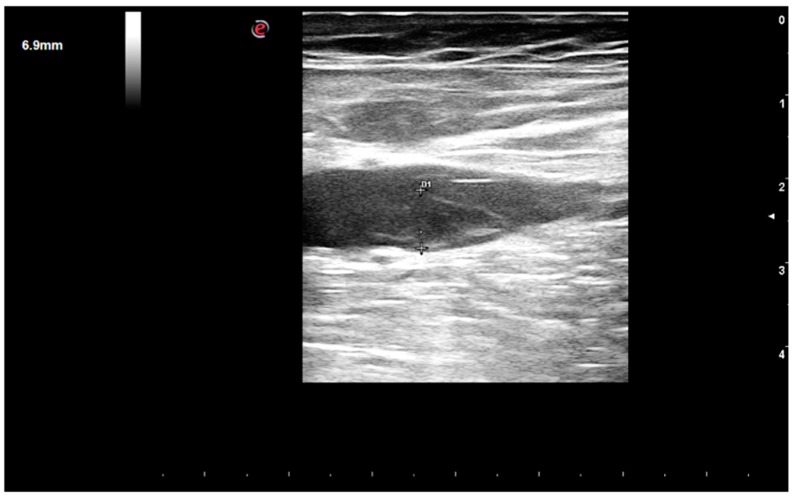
Ultrasonographic femoral scan. The exam showed in situ thrombosis of the left femoral vein which was not compressible with the probe.
